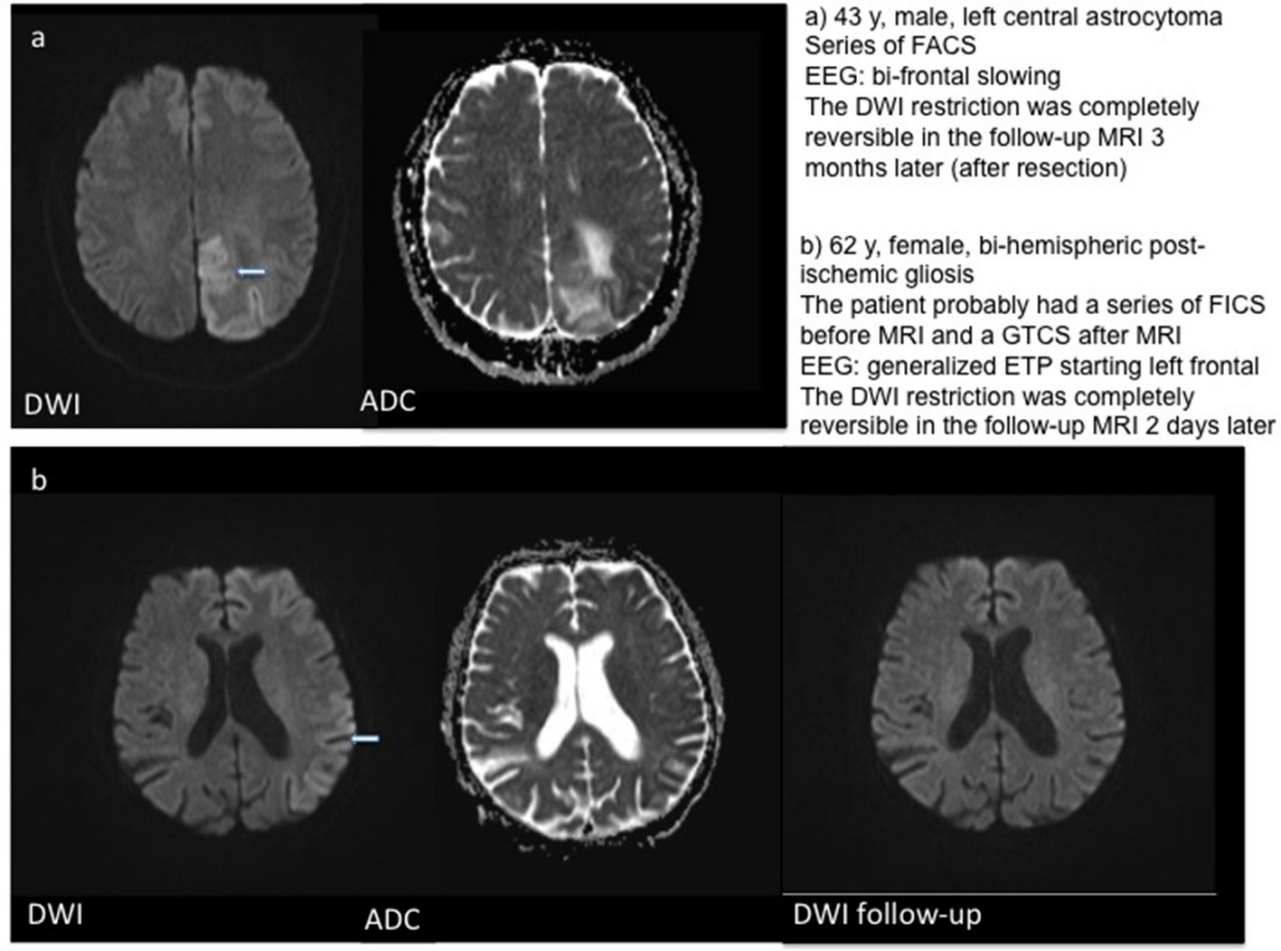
Examples for DWI restrictions in two patients with series of focal seizures. T2w–T2 weighted imaging.
